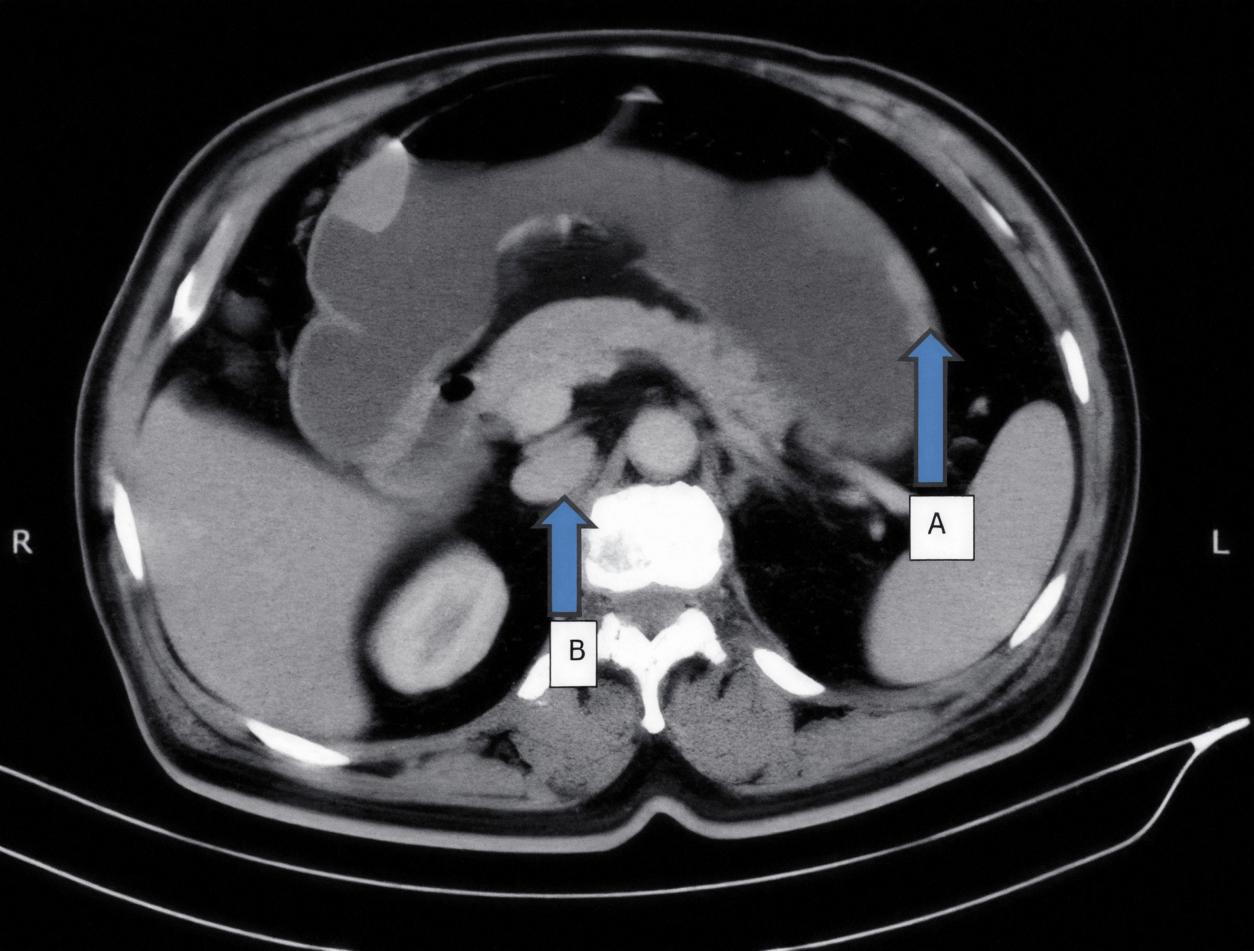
Contrast-enhanced CT of the abdomen revealing (A) heterogeneous enhanced polypoid lesions within the gastric antrum and fundus, and (B) a soft tissue mass lesion of 1.7 x 1.6 cm visualized in the retroperitoneal area.
